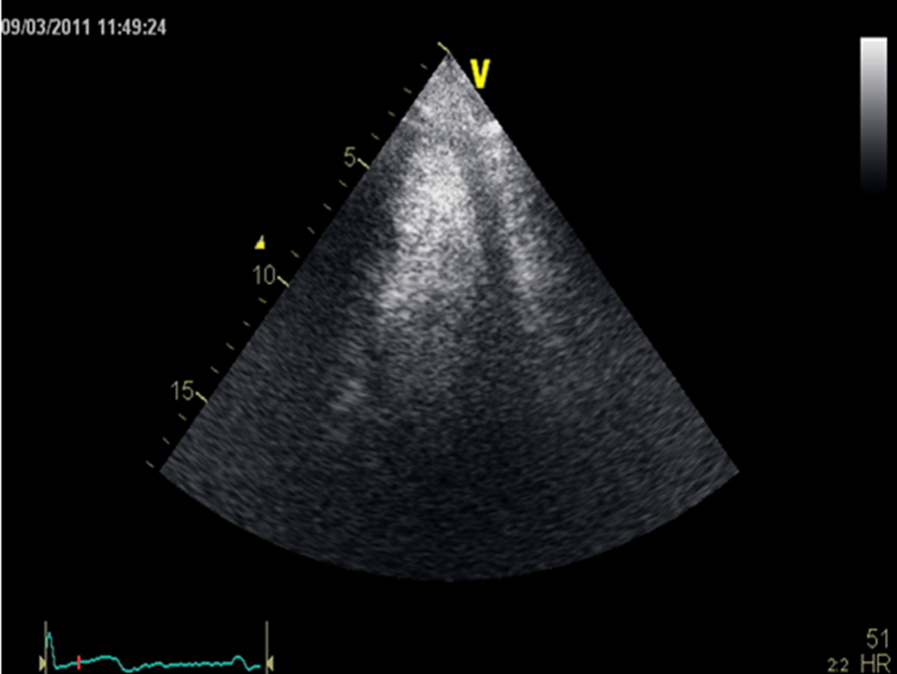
Constrast echocardiography performed one year after surgery showed a preserved LV systolic function, without evidence of a pseudoaneurysm.
